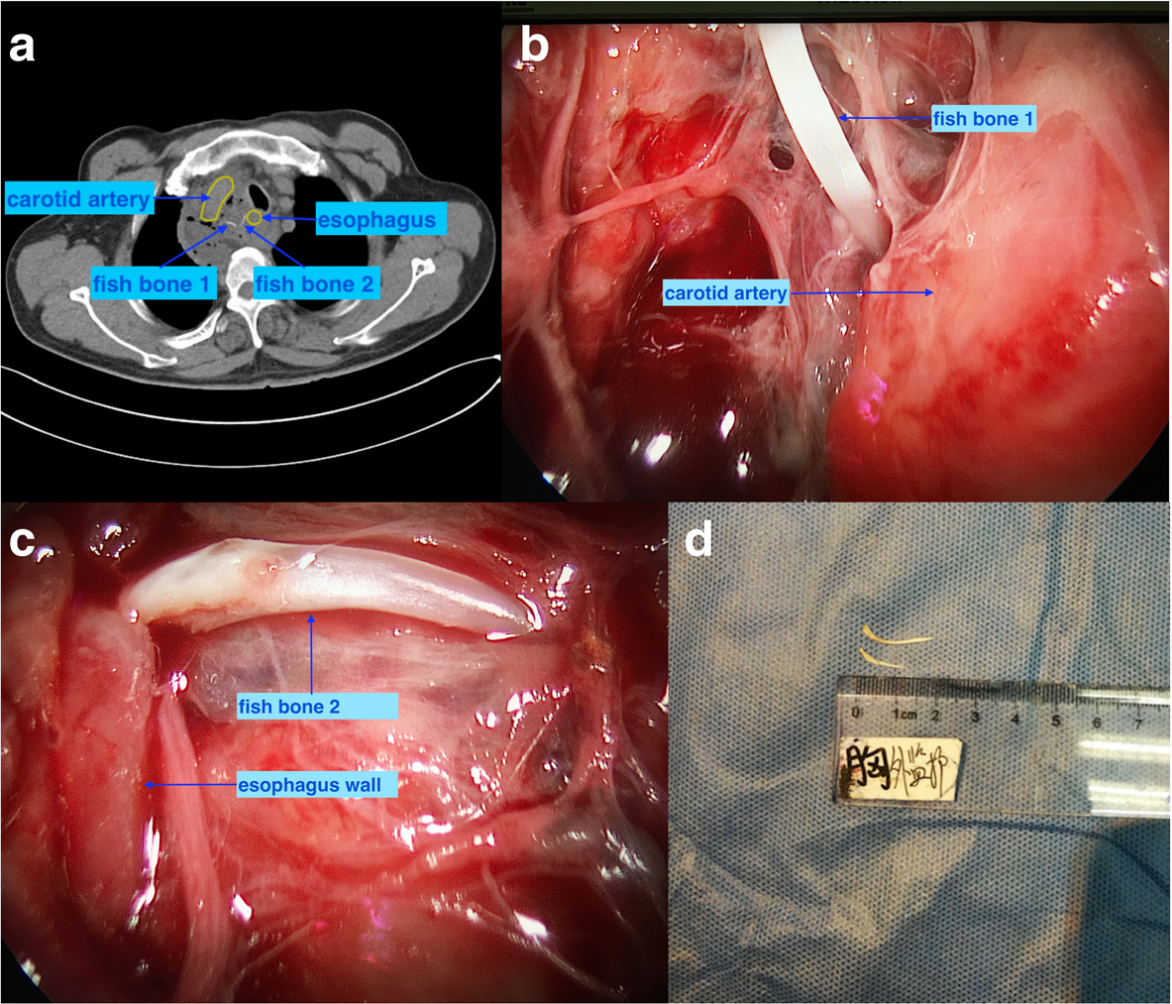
Preoperative, intraoperative and postoperative findings of fish bones. Preoperative, intraoperative and postoperative findings of fish bones. a CT result showed that an abscess confined to the right mediastinal pleural with two high density foreign bodies in the abscess at the level of the sternum angle. b Under video-mediastinoscopy, fish bone 1 was located at the medial margin of the carotid artery. c Under video-mediastinoscopy, fish bone 2 was located on the outside of the esophagus wall. d Two fish bones after being taken out
